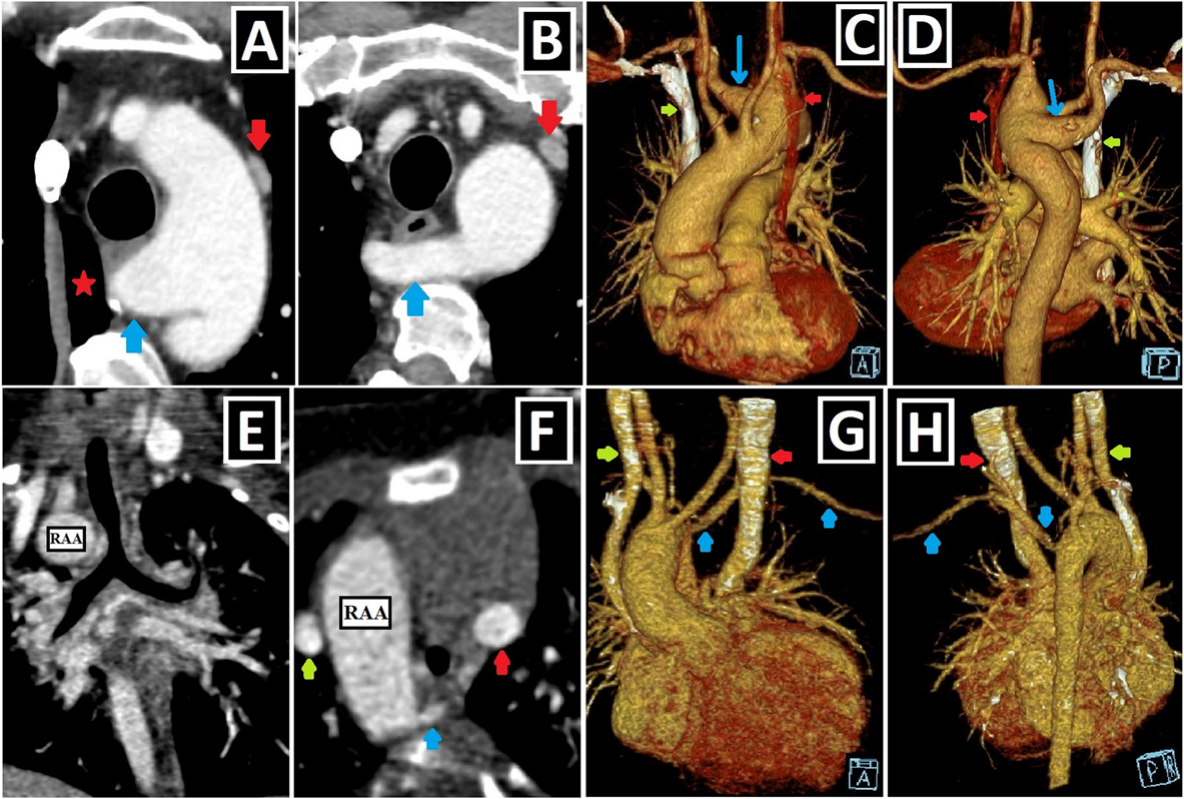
Aortic arch anomalies accompanying PLSVC-1. a Axial CT image depicts azygos lobe (red star), and ARSA with Kommerell diverticulum (blue arrow) in a patient with PLSVC (red arrow). b Axial CT image indicates ARSA (blue arrow) in a patient with PLSVC (red arrow). c, d 3D VRT images with anterior and posterior views depict ARSA with Kommerell diverticulum (blue arrows) in a patient with double SVC (green and red arrows). e–h Coronal-oblique reformatted (e), axial (f), and 3D VRT CT images with different views (g, h) show the right aortic arch (RAA) and ALSA (blue arrows) in a patient with double SVC (green and red arrows)
